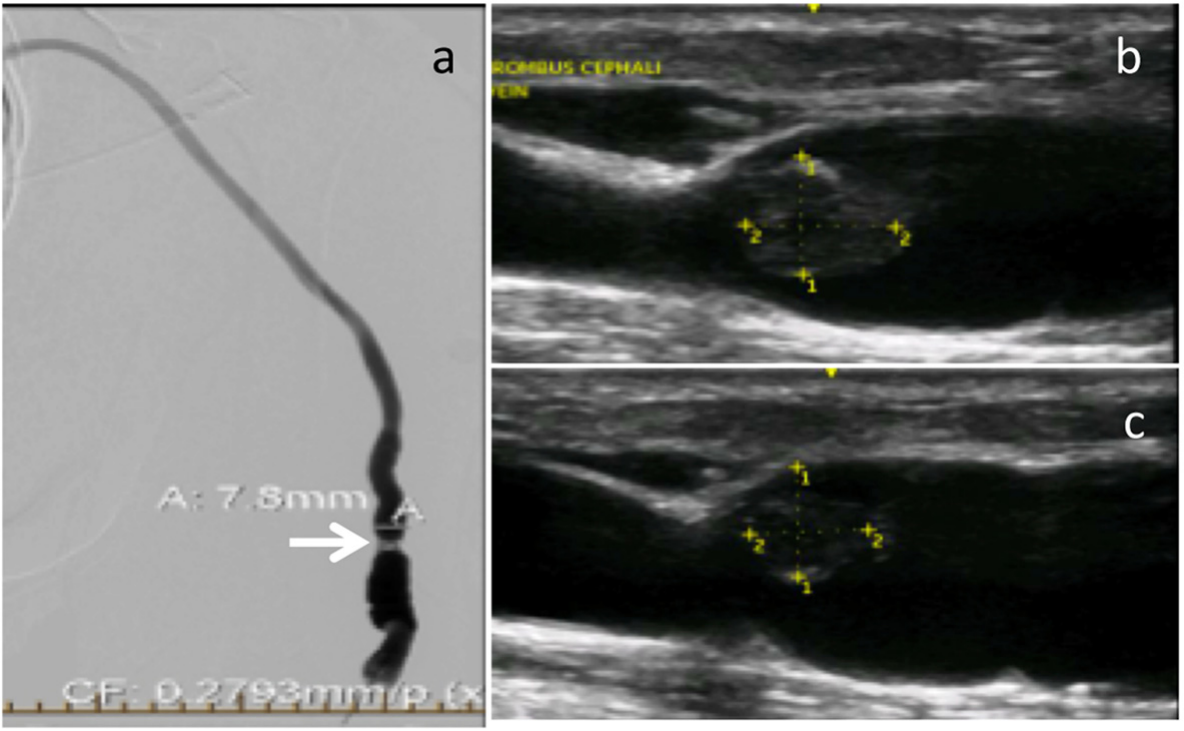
Fistulogram and ultrasound images of brachio-cephalic fistula. a: Fistulogram of Brachio-Cephalic AVF demonstrating a stenosis mid humerus (arrow). b: Doppler flow ultrasound of the AVF with large residual clot. c: Repeat ultrasound after tPA infusions resulted in decrease of clot burden.
